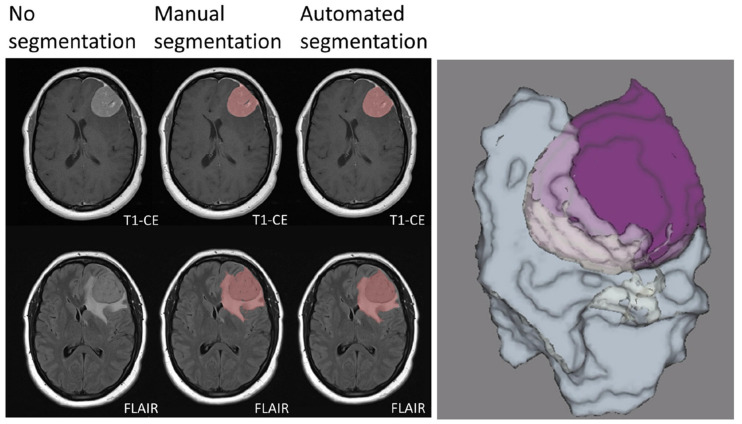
Manual and automated segmentation comparison in a meningioma of the left frontal convexity. The sharply demarcated lesion demonstrates intense contrast enhancement. Vasogenic edema of the surrounding white matter is also evident. Manual and automated segmentation are correctly matched. Three-dimensional rendering of the segmented tumor and edema volumes is also presented. T1-CE: contrast-enhanced T1-weighted imaging; FLAIR: fluid attenuated inversion recovery. Adapted from Ref. [13], under the terms of the Creative Commons Attribution-NonCommercial 4.0 License.
